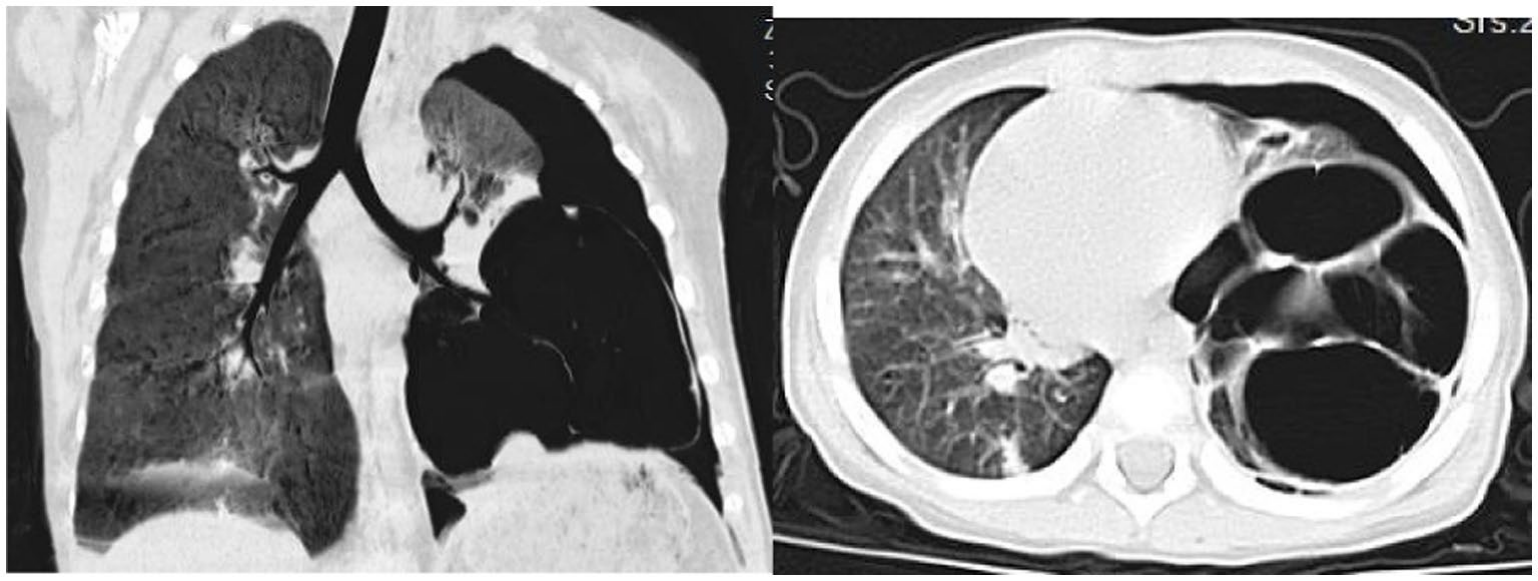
Chest CT scan on the 32th day of the illness.
